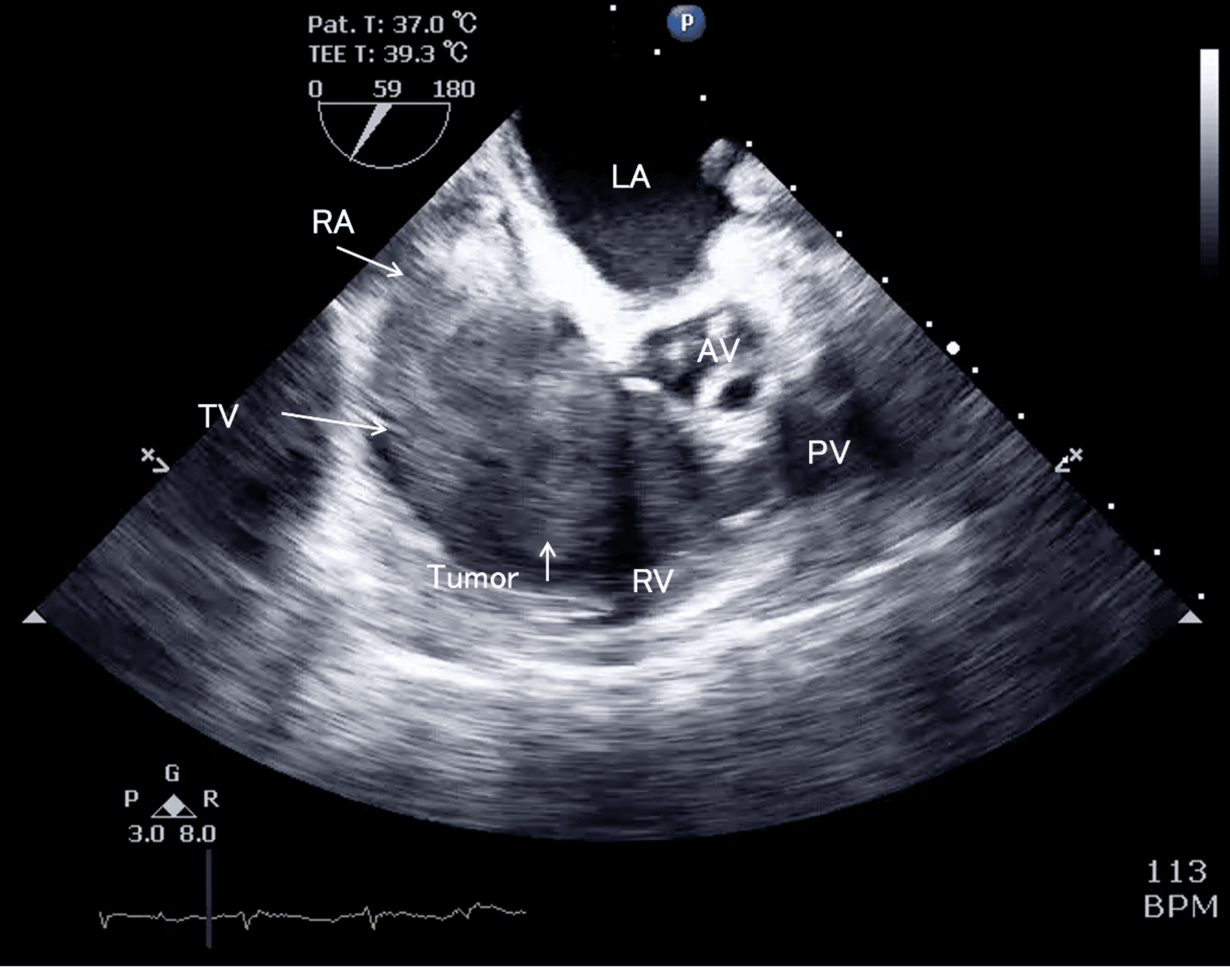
Intraoperative transesophageal echocardiography, mid-esophageal right ventricle inflow-outflow view As in Figure 1, the tumor was large enough to occupy the right atrium, with a spherical protrusion extending into the cardiac chamber that moved to and fro in synchrony with the cardiac cycle. During diastole, the tumor was entrapped in the tricuspid valve. Although the right ventricle was dilated, the right ventricular function was preserved. TV, tricuspid valve; RA, right atrium; RV, right ventricle; MV, mitral valve; LA, left atrium; LV, left ventricle; AV, aortic valve; PV, pulmonary valve
